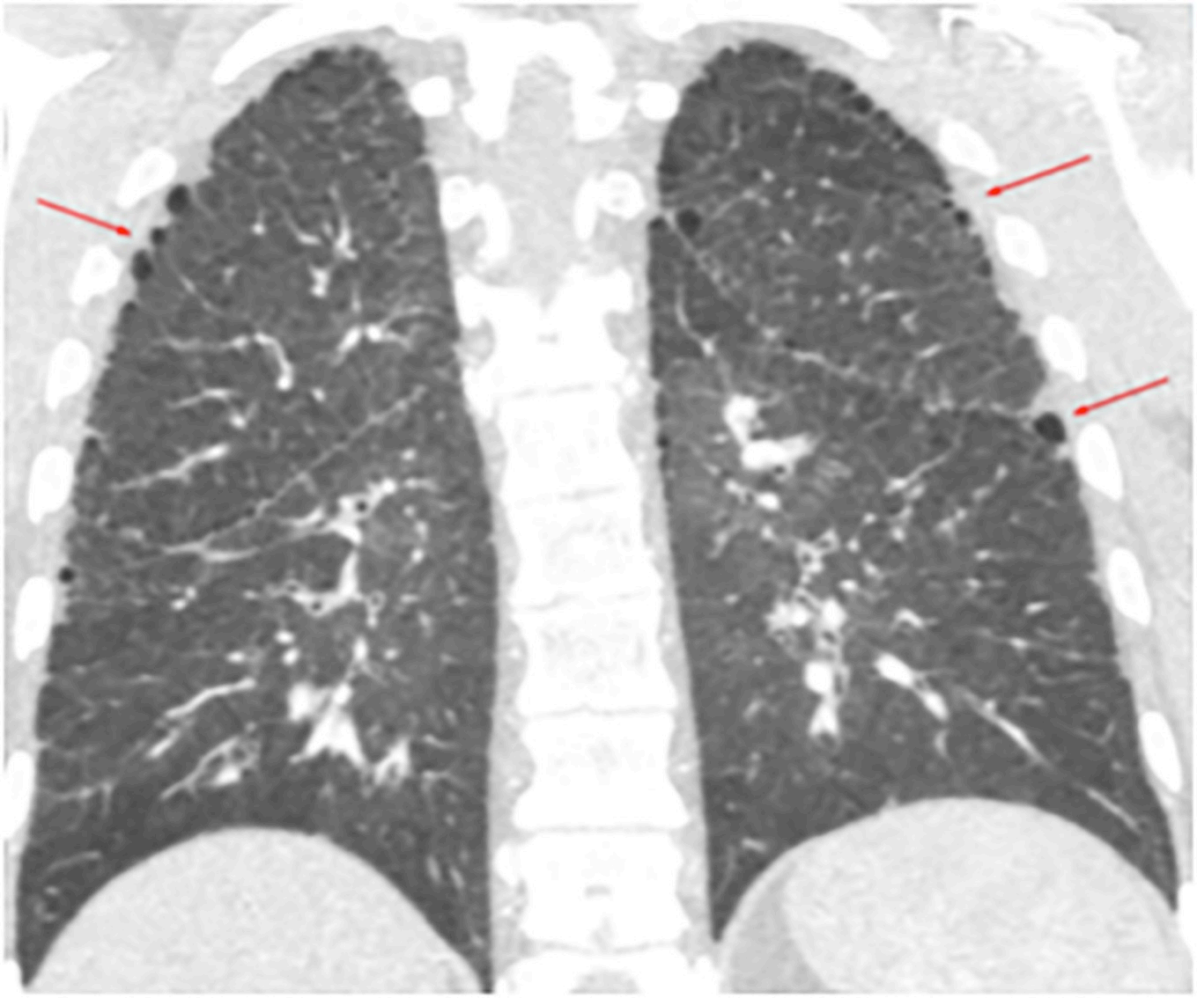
Patient 1 Multidetector Computed Tomography. Subtle emphysema radiological pattern with peripheral distribution (red arrows).
